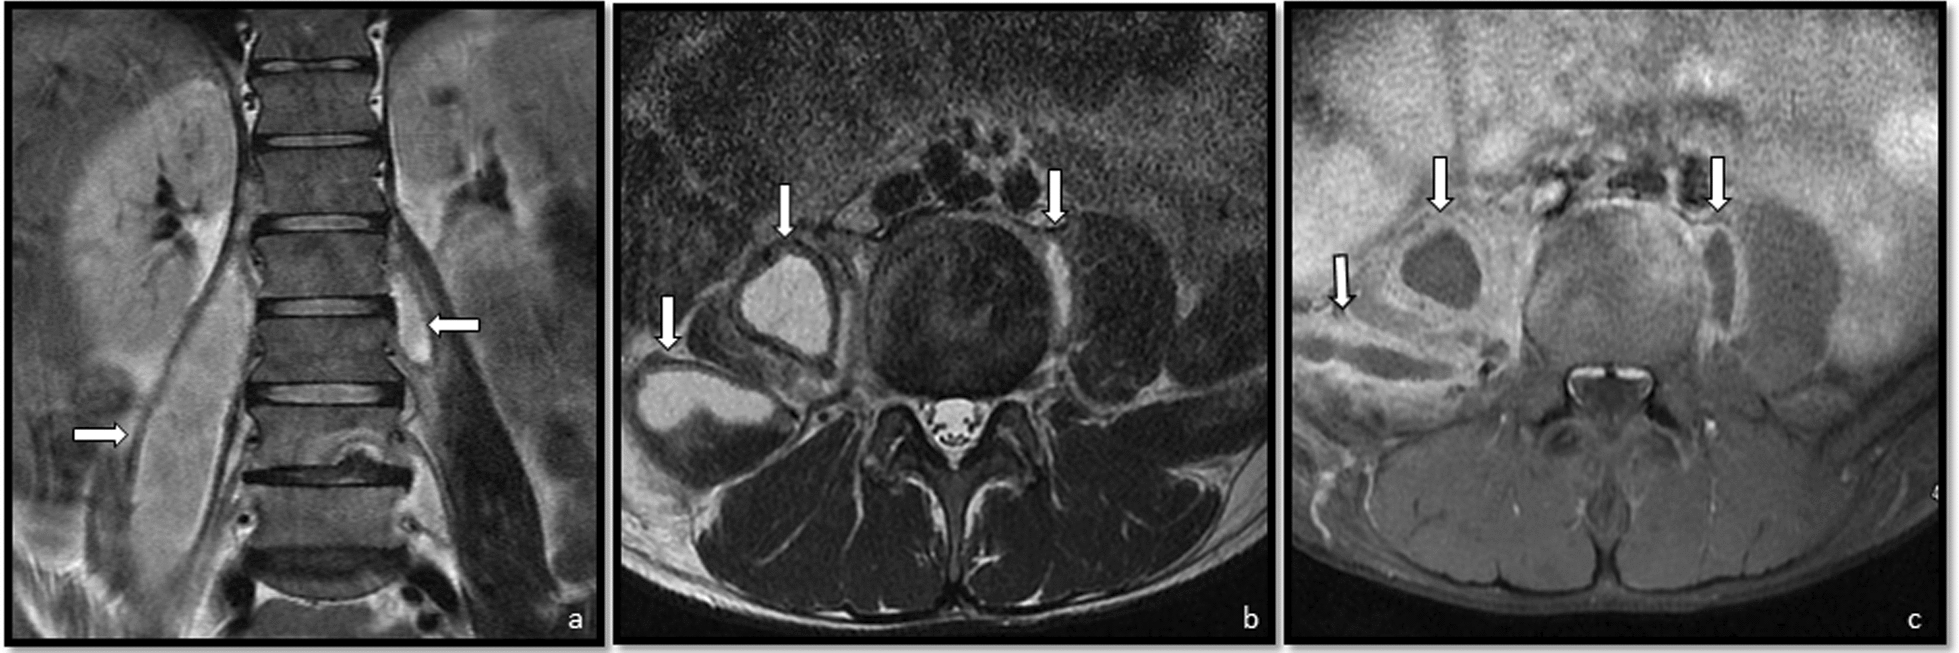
Fluid collection, hyperintense on T2-weighted images (a, b) and hypointense on T1-weighted images (c), consistent with abscess: on the right, located along the iliopsoas muscle, originating at the L1–L2 level and extending caudally to S2; on the left, involving the psoas major muscle between L2 and L3.
